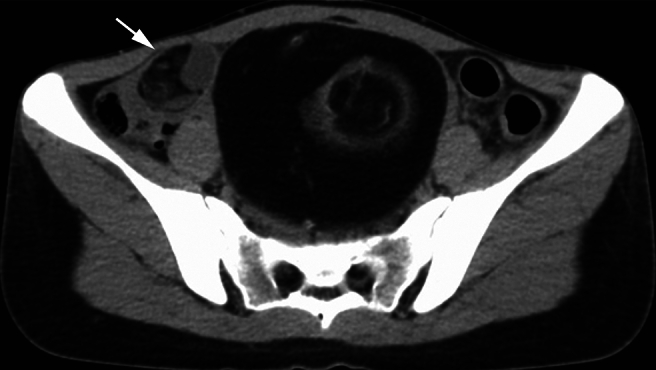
A non-contrast-enhanced computed tomography (CT) scan one year prior to laparoscopic surgery. An ovarian teratoma 10 cm long located in the middle of the pelvic cavity. The tumor contains a significant amount of fatty tissue and a hairball-like structure within the tumor. Another teratoma, 3 cm long, probably arising from the right ovary, is also observed in the same slice (arrow); however, the gynecologists who performed the former ovarian teratoma resection were not aware of the presence of the small teratoma arising from the contralateral ovary.
